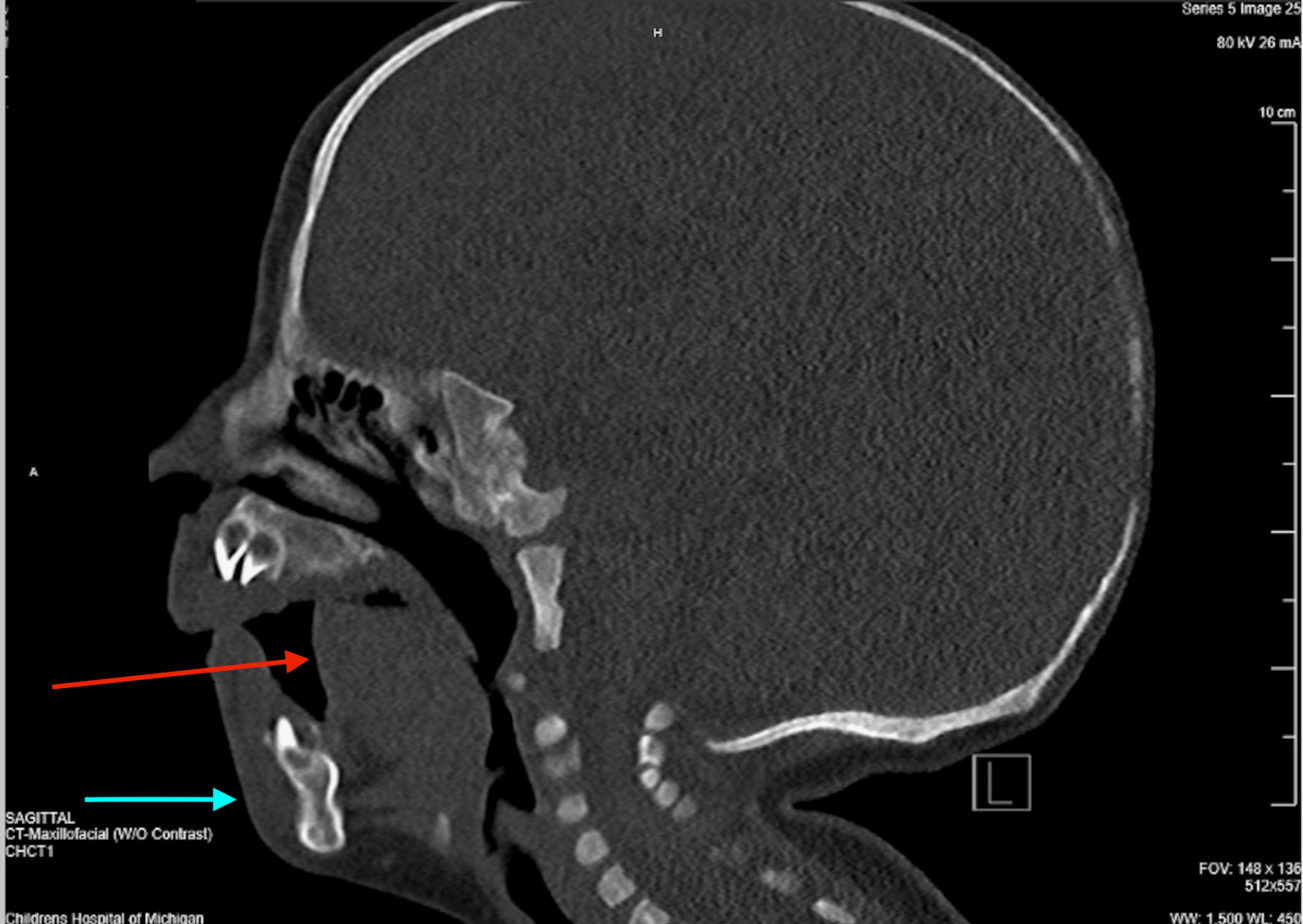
Sagittal view of the patient’s maxillofacial CT scan The image demonstrates the prominent micrognathia (blue arrow) and severe glossoptosis (red arrow) causing severe obstruction of the patient’s airway CT: computed tomography
